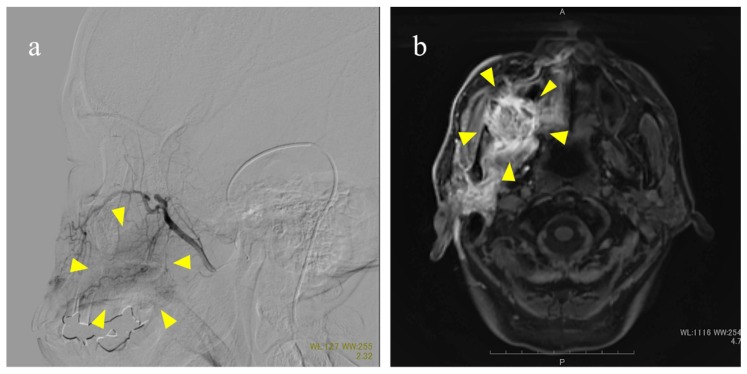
Methods to confirm flow using digital subtraction angiography (a) and magnetic resonance imaging (b). (a) The maxillary artery is displayed. Arrows indicate the labeled maxillary gingival tumor; (b) Perfusion of the right maxillary gingiva, palate, pterygoid muscle, and parotid gland. Arrows indicate the enhanced tumor. Scale bar: 10mm.
